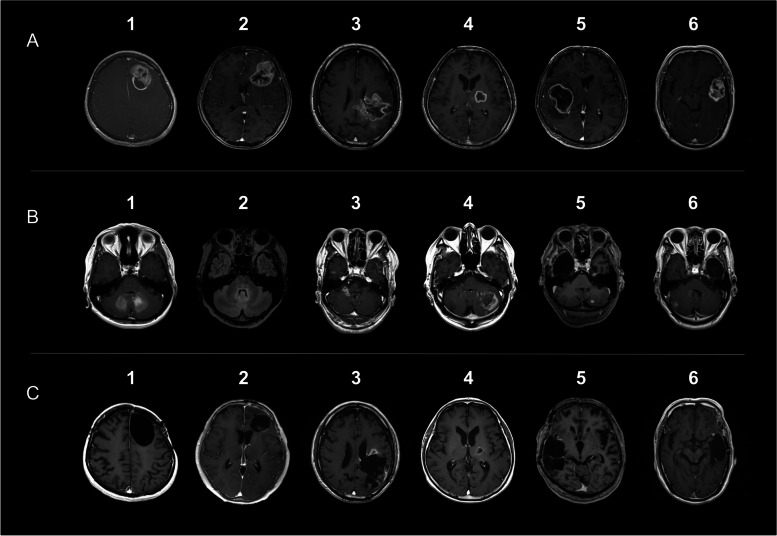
Axial gadolinium-enhanced T1-weighted images of patients with infratentorial recurrence (ITR). A Representative images of the initial tumors. B Representative images of the ITR. Image of the patient 2 is fluid-attenuated inversion recovery images. C Images of the cavities of the initial tumors at ITR development
